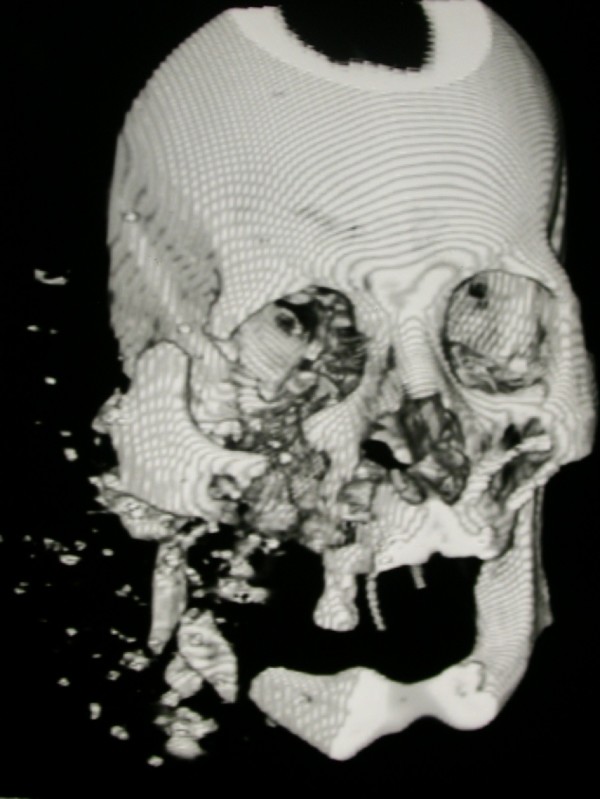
A patient with high velocity long distance injury, with severe soft tissue damage of the right chick. 3 dimensions CT shows comminuted fracture of the right orbit, zygoma and right mandible.
